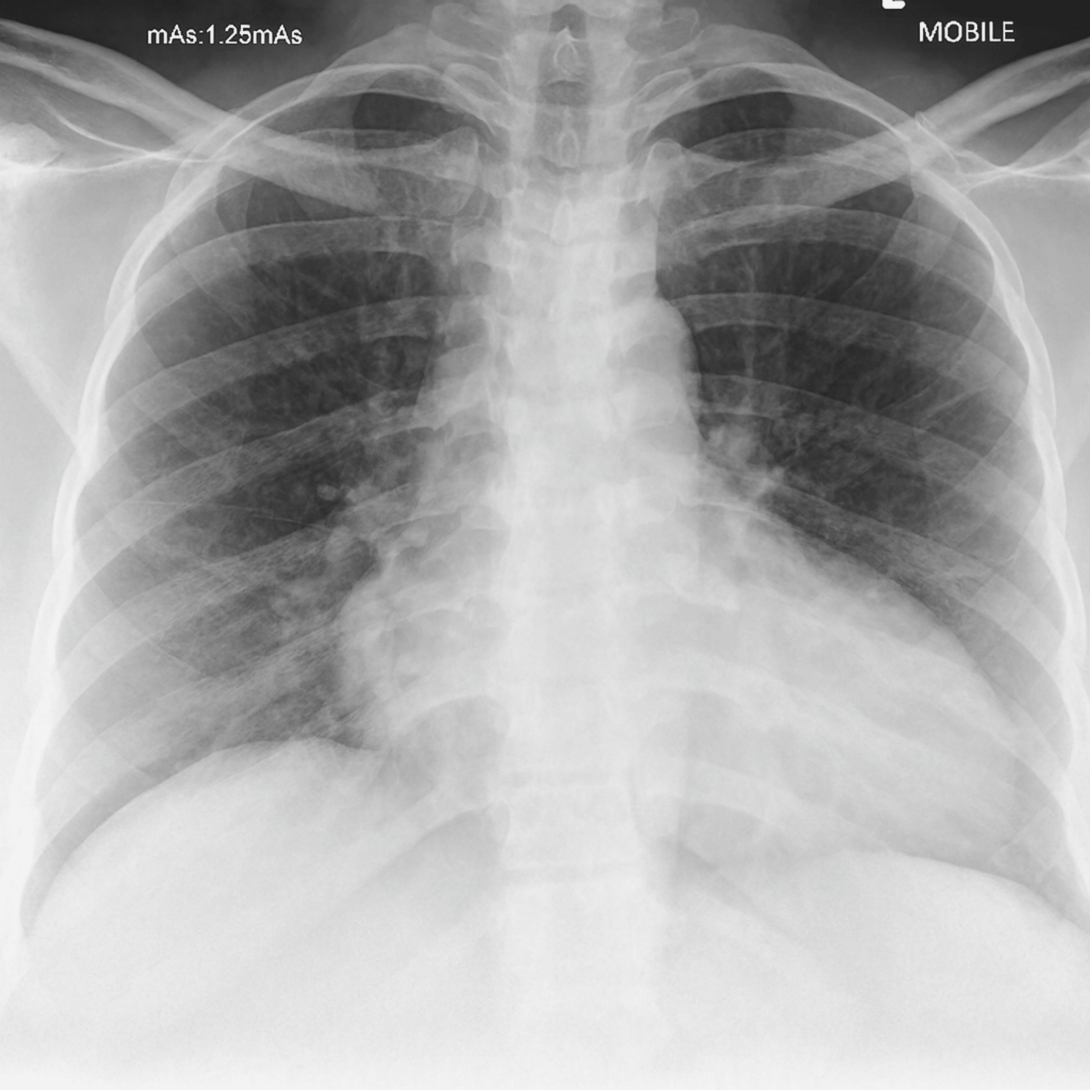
Chest X-ray showing pulmonary congestion with bilateral prominent vascular markings.
